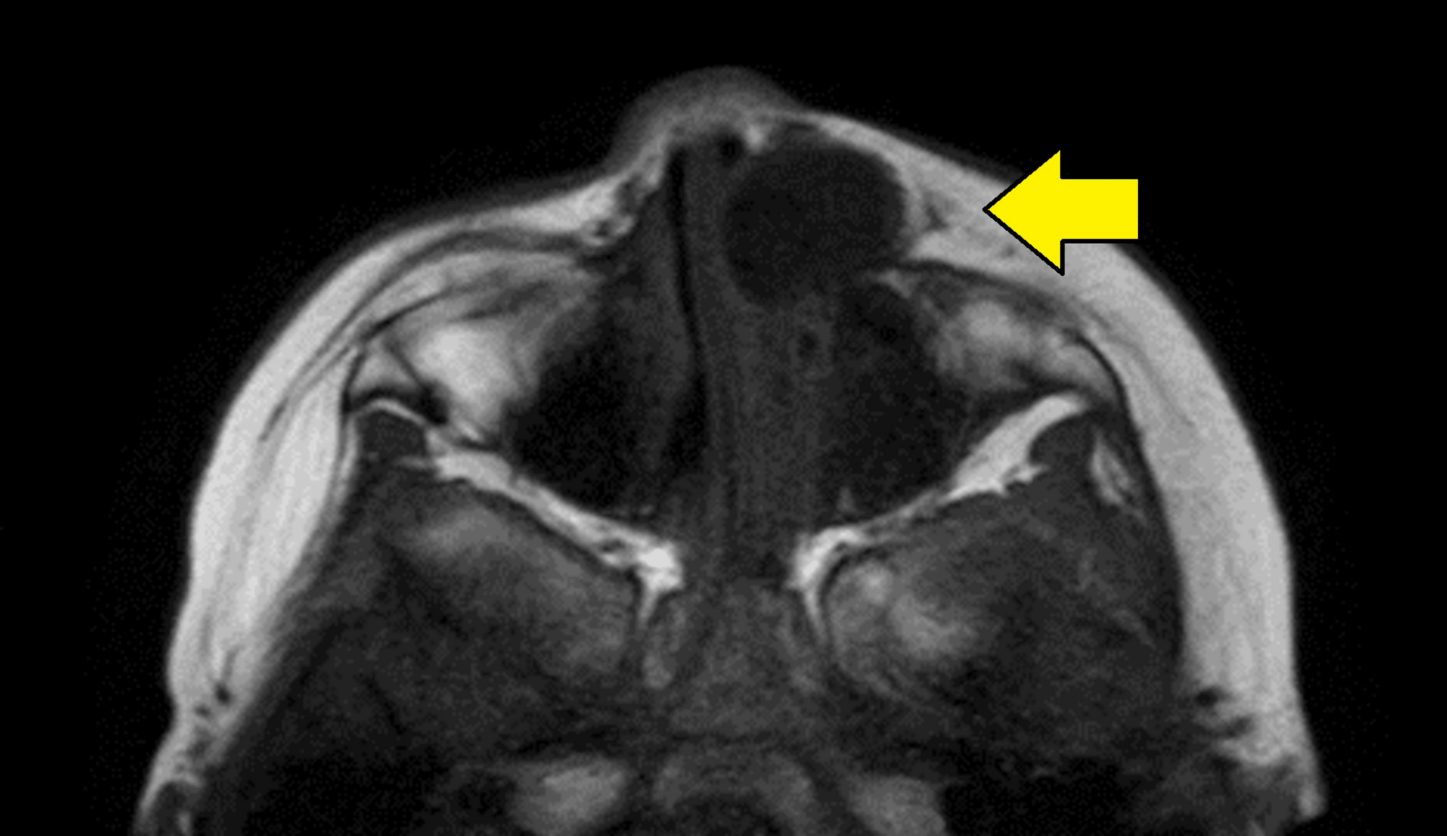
T1-weighted image showing a cystic lesion with low signal intensity.
